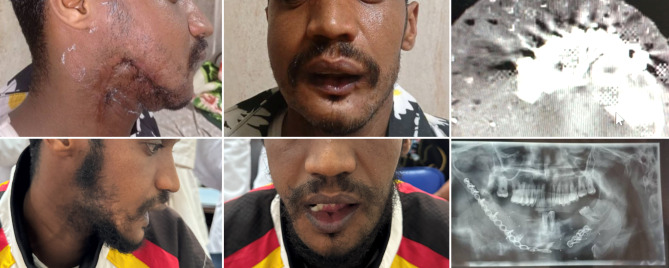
Case 2: secondary mandibula reconstruction after gunshot injury. a, b Preoperative clinical photograph. c Preoperative CT scan of the mandible demonstrating the segmental defect. d, e Postoperative clinical photograph. f Postoperative X-ray confirming fixation of the fibula flap with reconstruction plate and restoration of mandibular continuity
